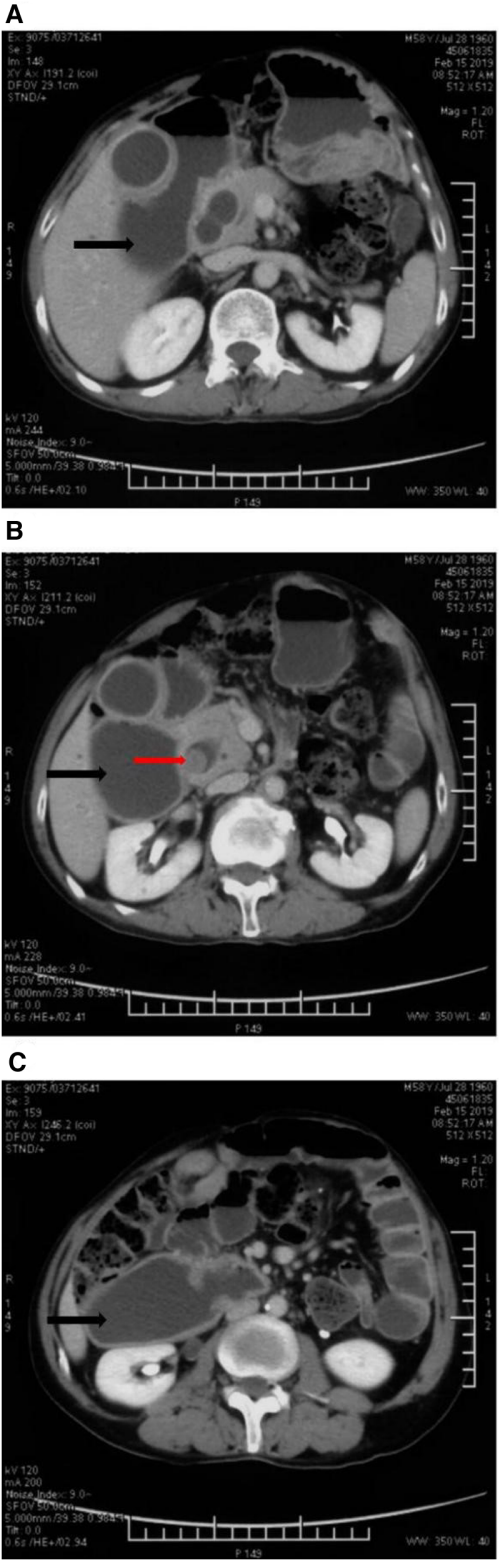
Abdominal enhanced CT images: the dilated duodenum was indicated by black arrows in (A), (B) and (C). The stone in the dilated common bile duct was marked with a red arrow in (B).
